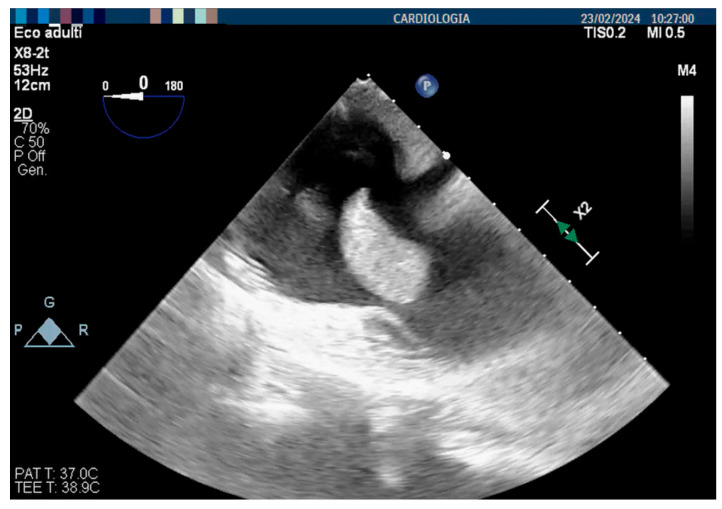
Pre-procedure transesophageal echocardiogram (TEE) showing a thrombus attached to the right atrium free wall.
